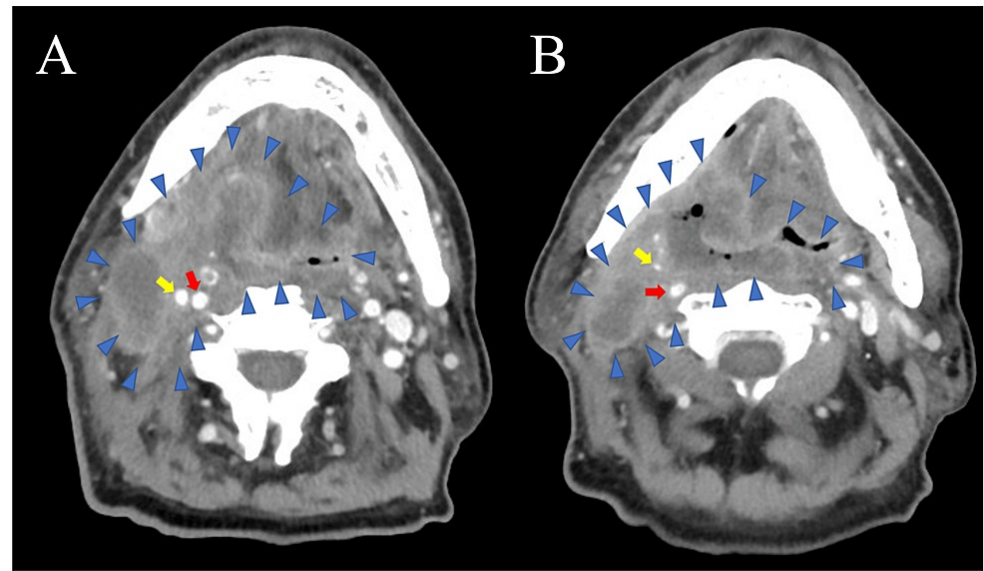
Contrast-enhanced computed tomography in Case 1. (A) Primary tumor enlargement near the right ECA (yellow arrow), and the center of the tumor shows hypodensity. (B) Tumor invasion of the right ECA (yellow arrow). The tumor is surrounded by blue arrowheads. Red arrows indicate the internal carotid arteries and yellow arrows indicate the ECA ECA: external carotid artery
